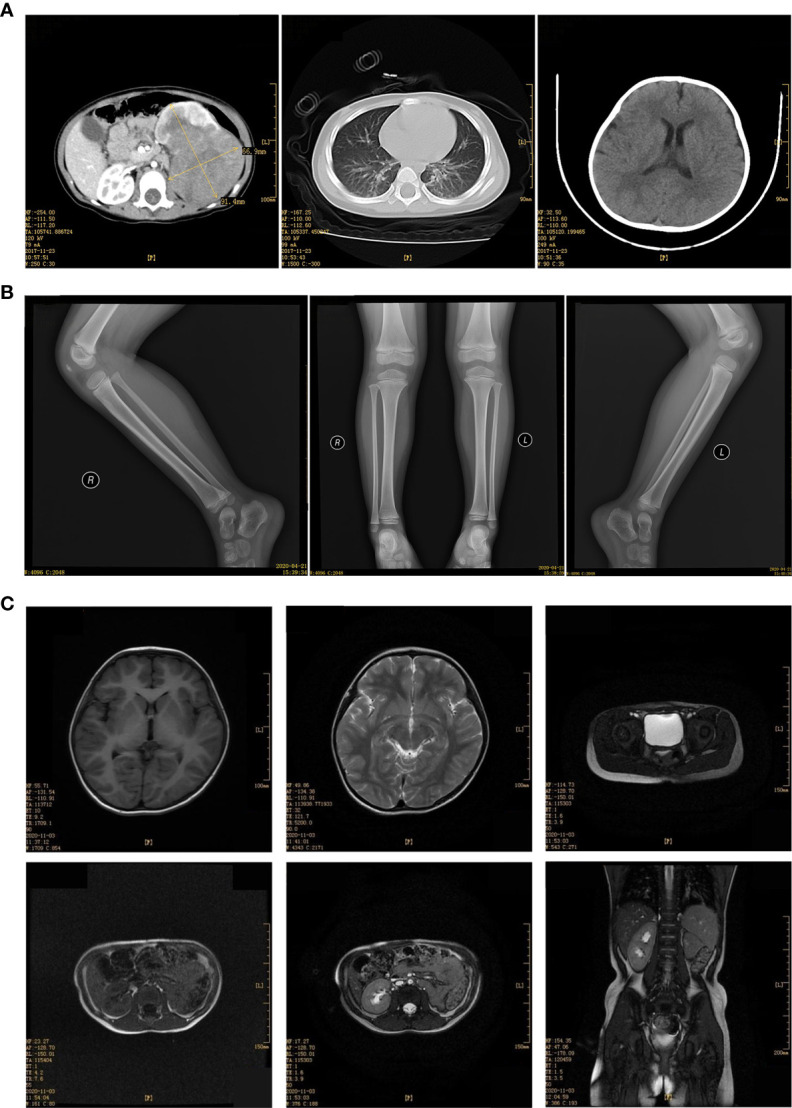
(A) Enhancement of CT plain scan of chest and abdomen before the operation (December 29, 2017). (B) Bilateral hip joint X tablets after the operation (April 21,2020). (C) MRI scan of the head, chest, abdomen, and pelvis after the operation (December 07, 2020).
